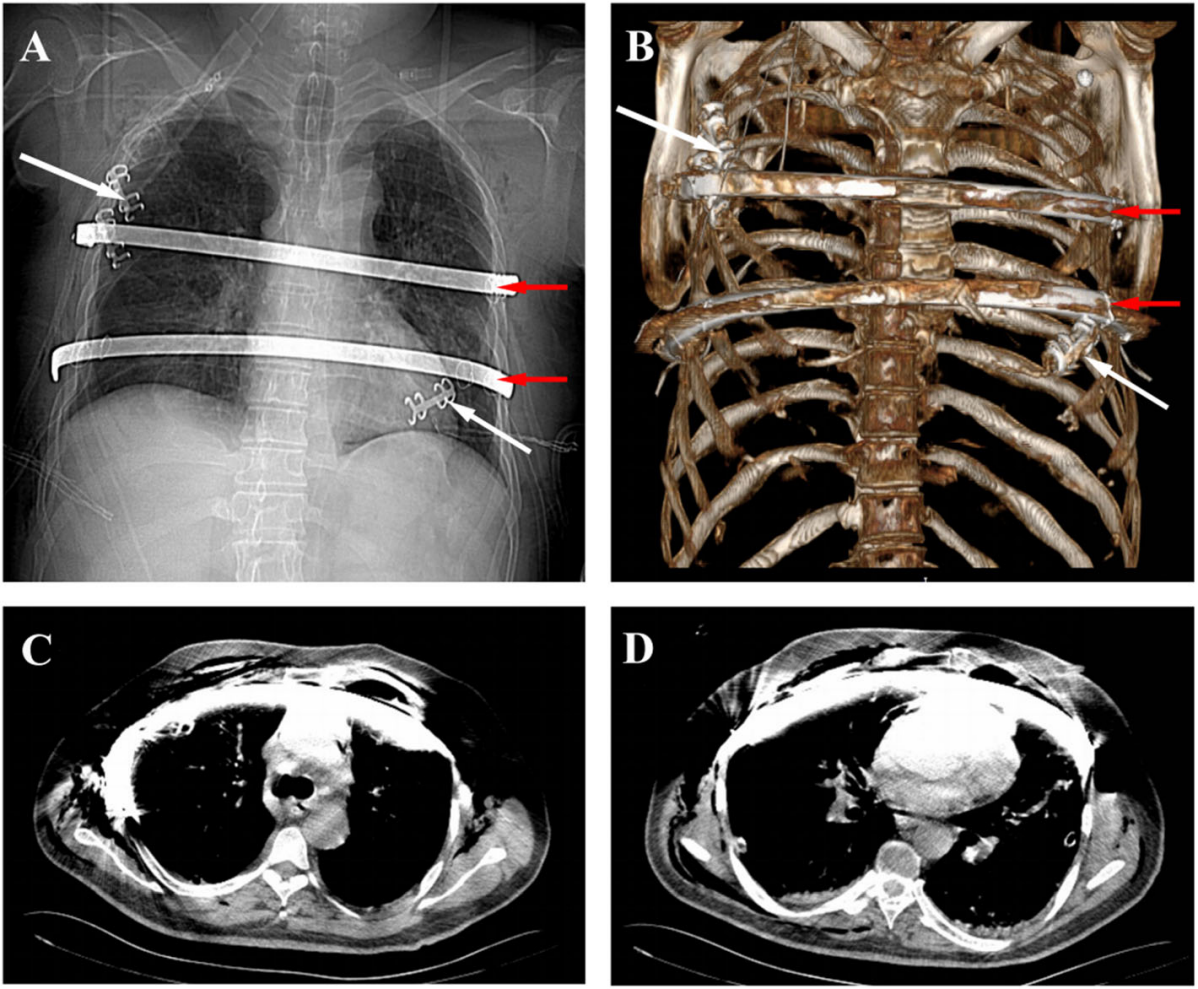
postoperative chest wall a postoperative chest X-ray with rib fixation plates (white arrows) and Nuss bars (red arrows) in place. b postoperative bone three-dimensional reconstruction of the chest wall with rib fixation plates (white arrows) and Nuss bars (red arrows) in place. c upper bar in the third intercostal space d lower bar infigure the fifth intercostal space
